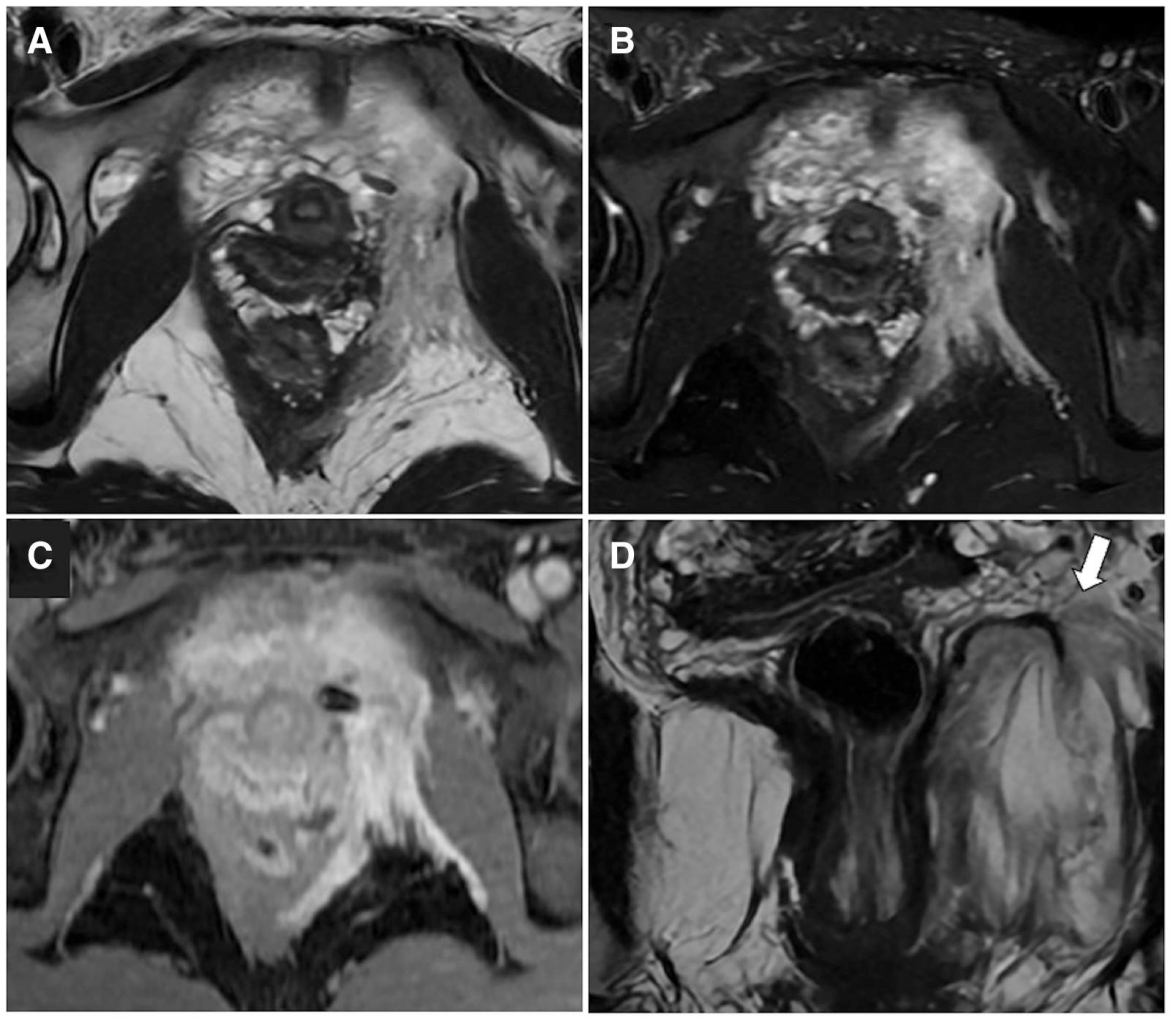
Aggressive angiomyxoma. MRI shows a solid mass with laminated appearance centred in the left pelvis, hyperintense on T2-WI (A). The lesion is more prominent on fat-saturated images (B) and shows vivid contrast enhancement (C). The characteristic translevator extension is visible on coronal images (arrow in D). Abbreviation: WI = weighted imaging.
